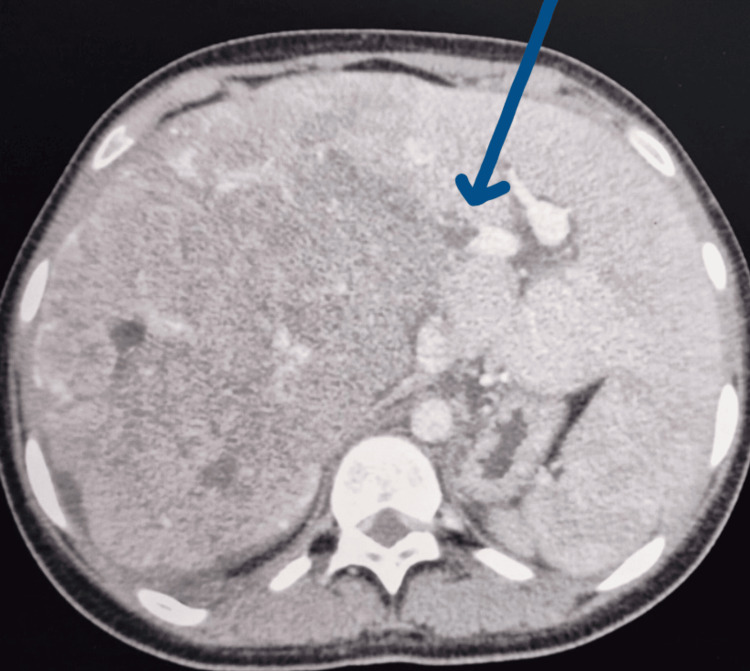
Contrast-enhanced computed tomography showing diffusely enlarged heterogeneous liver involving the whole of the right lobe with peripherally enhancing lobulated components and internal cystic areas with a mass displacing the IVC, and an arrow showing right portal vein thrombus pre-neoadjuvant chemotherapy IVC: inferior vena cava
